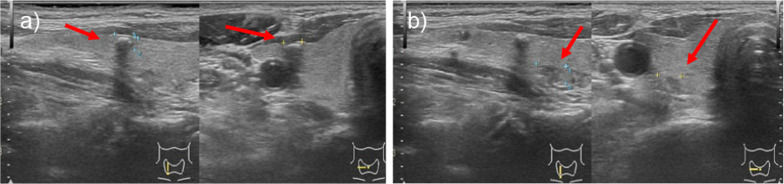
Ultrasonographic findings of the thyroid tumors. a A hypoechoic mass with rim calcification, 4 mm in size, is found in the middle of the right lobe of the thyroid gland. b An irregular hypoechoic mass, 6 mm in size, is found in the middle of the right lobe of the thyroid gland
